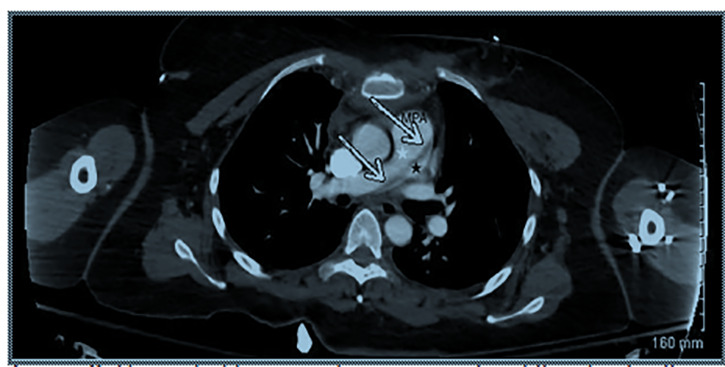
Computed tomography angiography of the chest with intravenous contrast in axial view showing displacement of the left atrium (arrows). True (white star) and false (black star) lumens can also be visualized.
